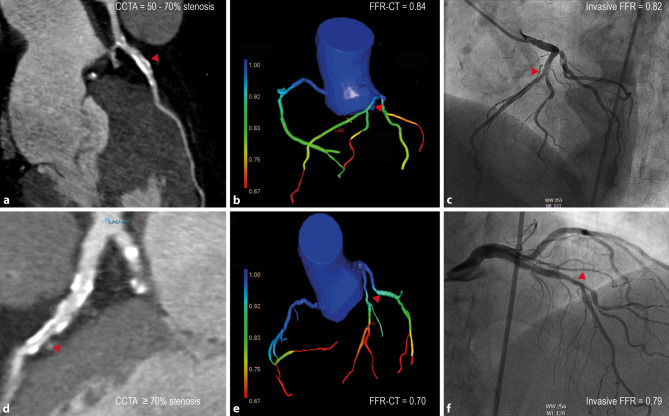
a–f Example of coronary computed tomography angiography (*CCTA*), computed tomography-derived fractional flow reserve (*CT-FFR*) and invasive FFR in two study patients. a CCTA demonstrates a 50–70% obstructive stenosis of the mid-segment of the left anterior descending artery (LAD) and therefore a significant stenosis. b The CT-FFR algorithm computes an FFR of 0.84, indicating non-significant vessel ischaemia. c Invasive FFR measurement demonstrates obstructive stenosis and an FFR value of 0.82, indicating no vessel ischaemia. d The calcified stenosis in the mid-LAD is reduced by more than 70%. e CT-FFR indicates the stenosis to be significant with an FFR value of 0.70. f Invasive FFR confirms the findings of CCTA and CT-FFR. An FFR of 0.79 is measured, indicating haemodynamically significant coronary artery disease
